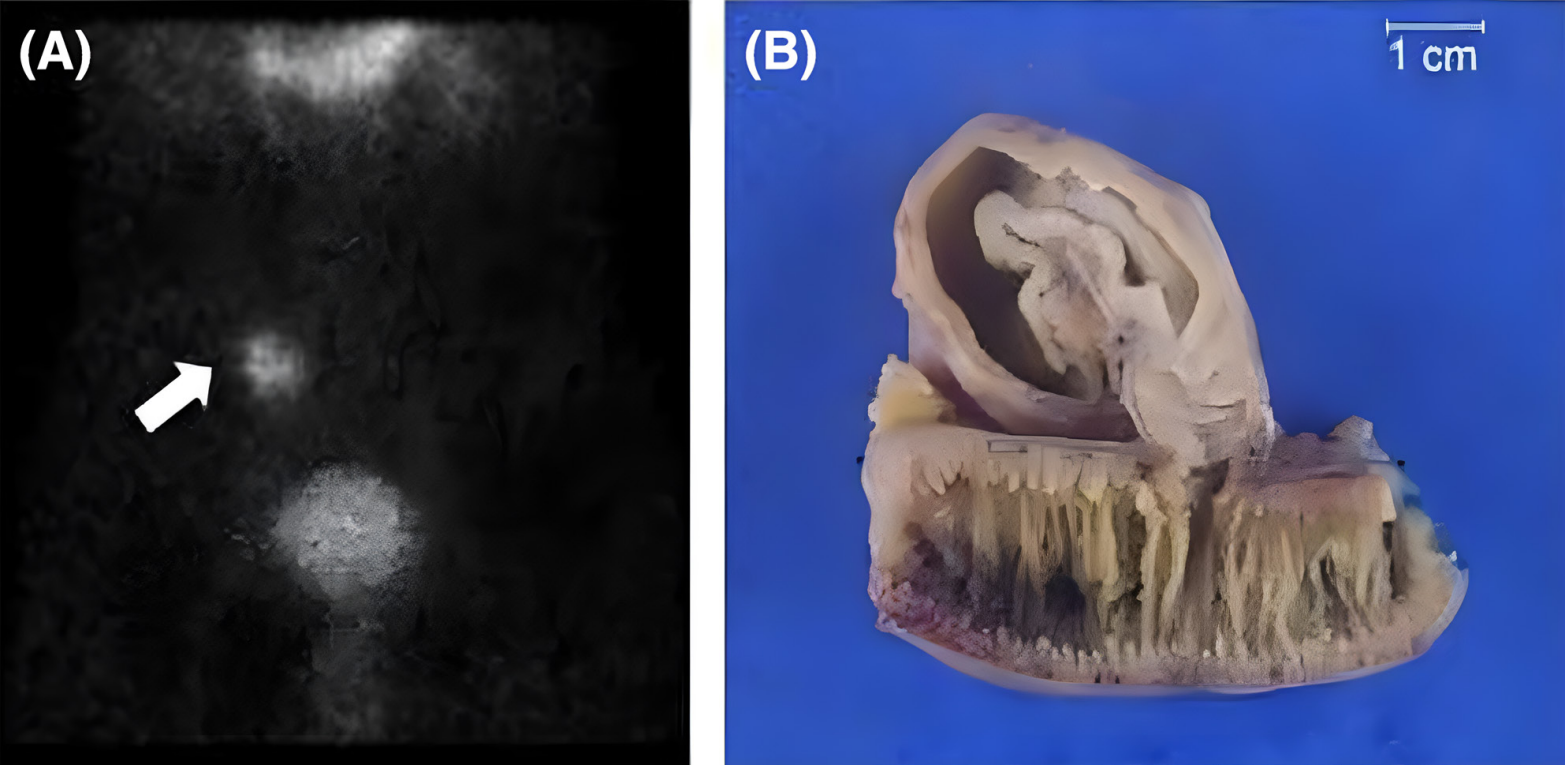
Technentium‐99m pertechnetate scintigraphy (A) indicating bleeding Meckel's diverticulum. Segmental resection of ileum with Meckel's diverticulum (B).
